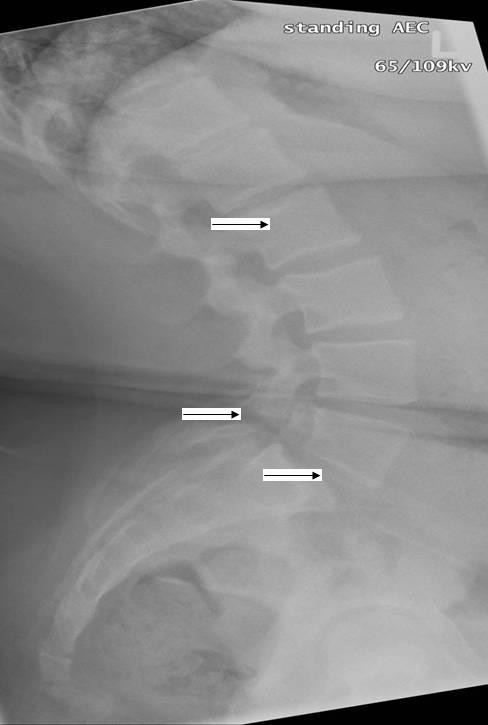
Lateral radiograph of the lumbosacral spine in a 12 year old obese girl with low back pain. Bilateral pars defects (middle arrow) are present and there is Grade I spondylolisthesis (bottom arrow) of L5 on S1. There is also evidence of Schmorl's nodes (top arrow) within the lower thoracic spine. (Radiograph courtesy of BC Children's Hospital).
